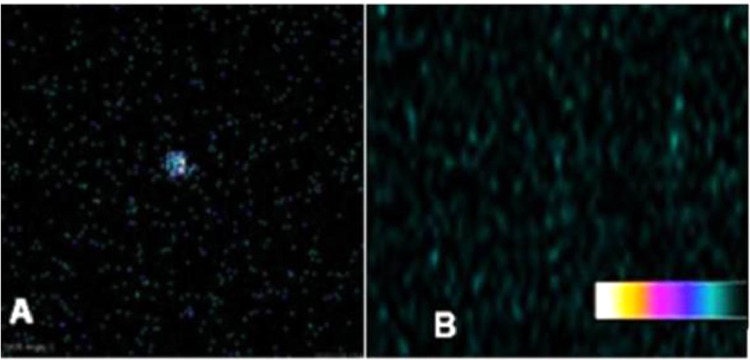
Ex vivo graft vs. biceps femoris muscle ^99m^Tc-biodistribution. 10-minute ^99m^Tc-planar scintigraphy biodistribution on the 14th day revealing (A) excinded thyroid graft with radionuclide accumulation. (B) Previously attached graft region, biceps femoris muscle, after graft excision with no radioactive emission.
